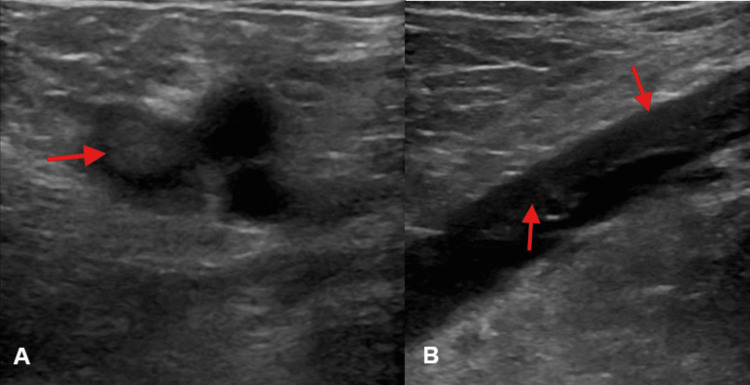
Ultrasound images (A) Deep vein thrombosis (red arrow) on ultrasound of the left common femoral vein at the saphenofemoral junction; (B) Deep vein thrombosis (red arrows) on sagittal view ultrasound of the left popliteal vein.
